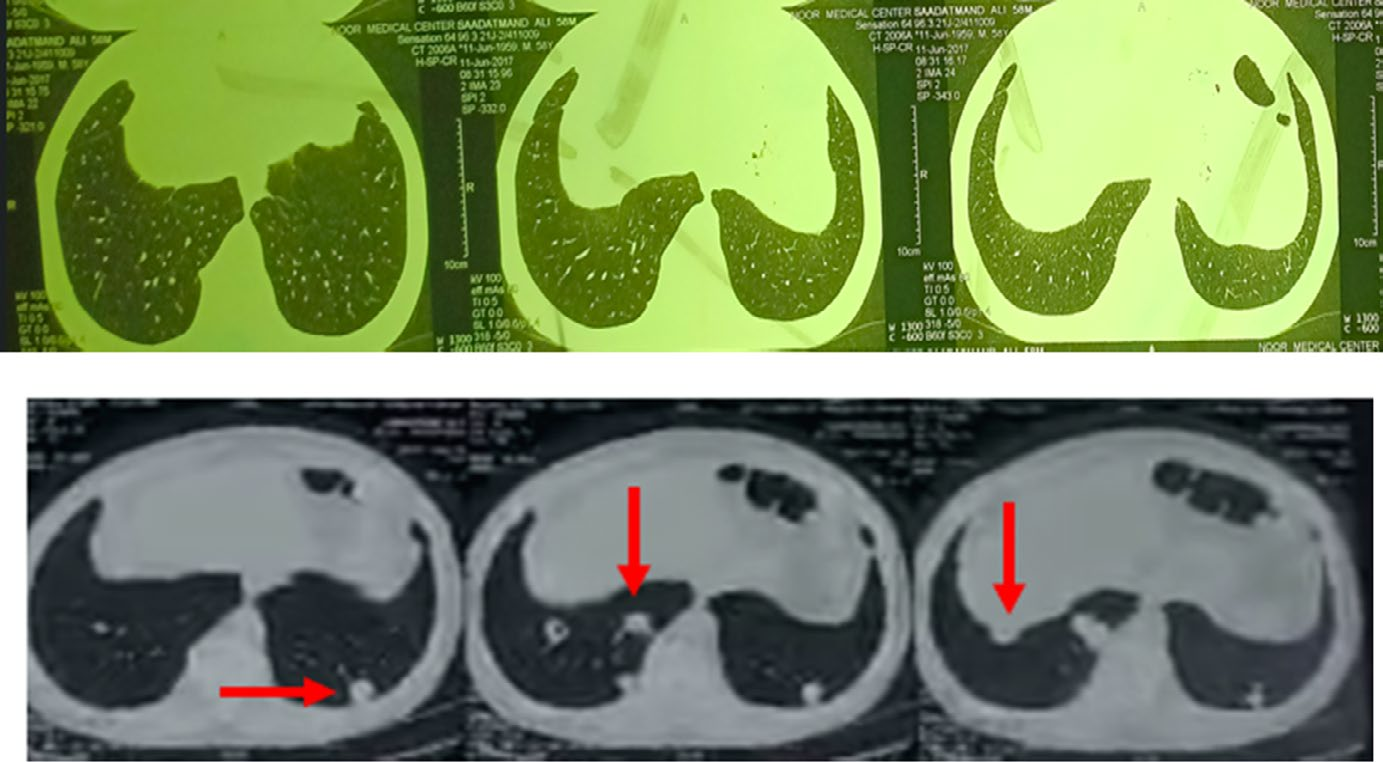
Chest CT axial images, Upper panel, before leflunomide therapy. Lower panel, after leflunomide therapy, showing multiple rheumatoid nodules.
